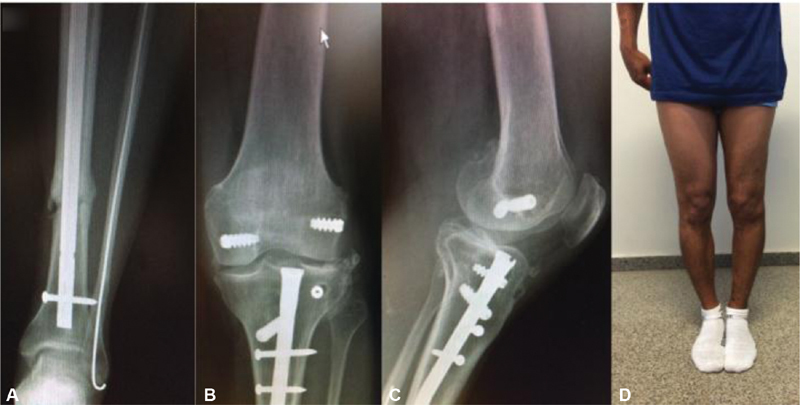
Follow-up images at 1 year with plain radiographs of the left leg (
